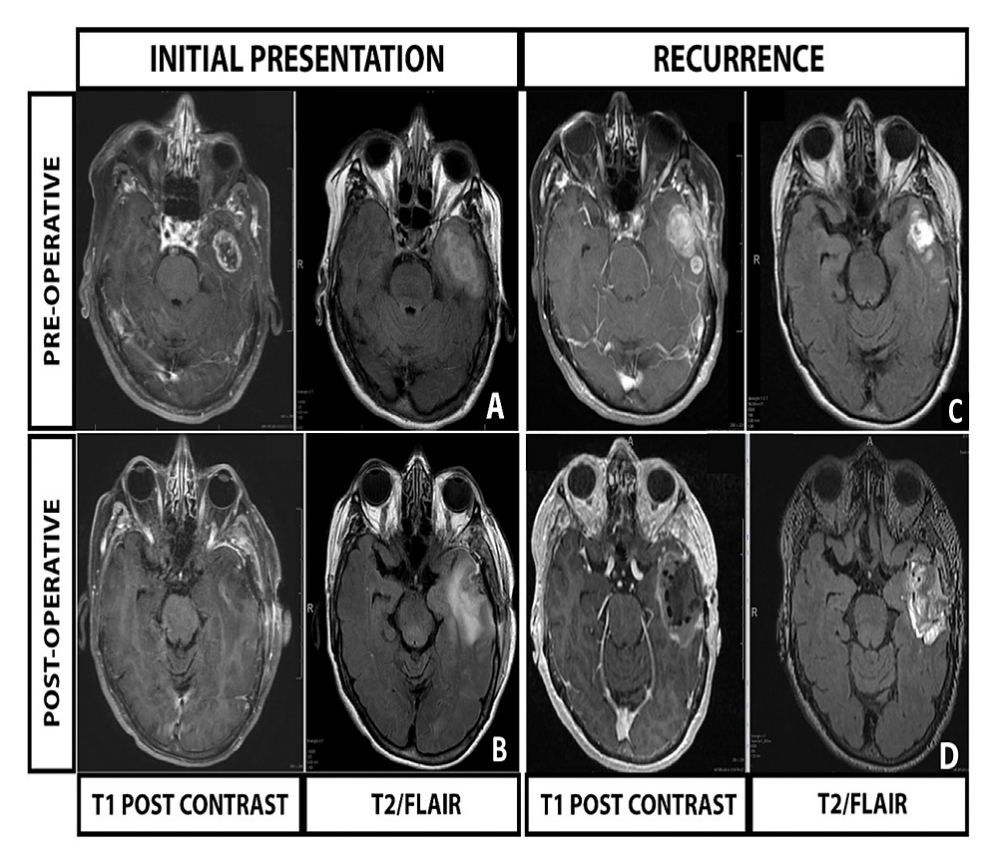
Representative imaging for the patient’s index presentation and recurrence (A) Post-contrast and T2/FLAIR imaging at presentation, (B) Initial post-operative, post-contrast and T2/FLAIR imaging demonstrating maximal safe resection (GTR), (C) Post-contrast and T2/FLAIR imaging at recurrence, and (D) Recurrence post-operative imaging, post-contrast and T2/FLAIR with GT placement.
